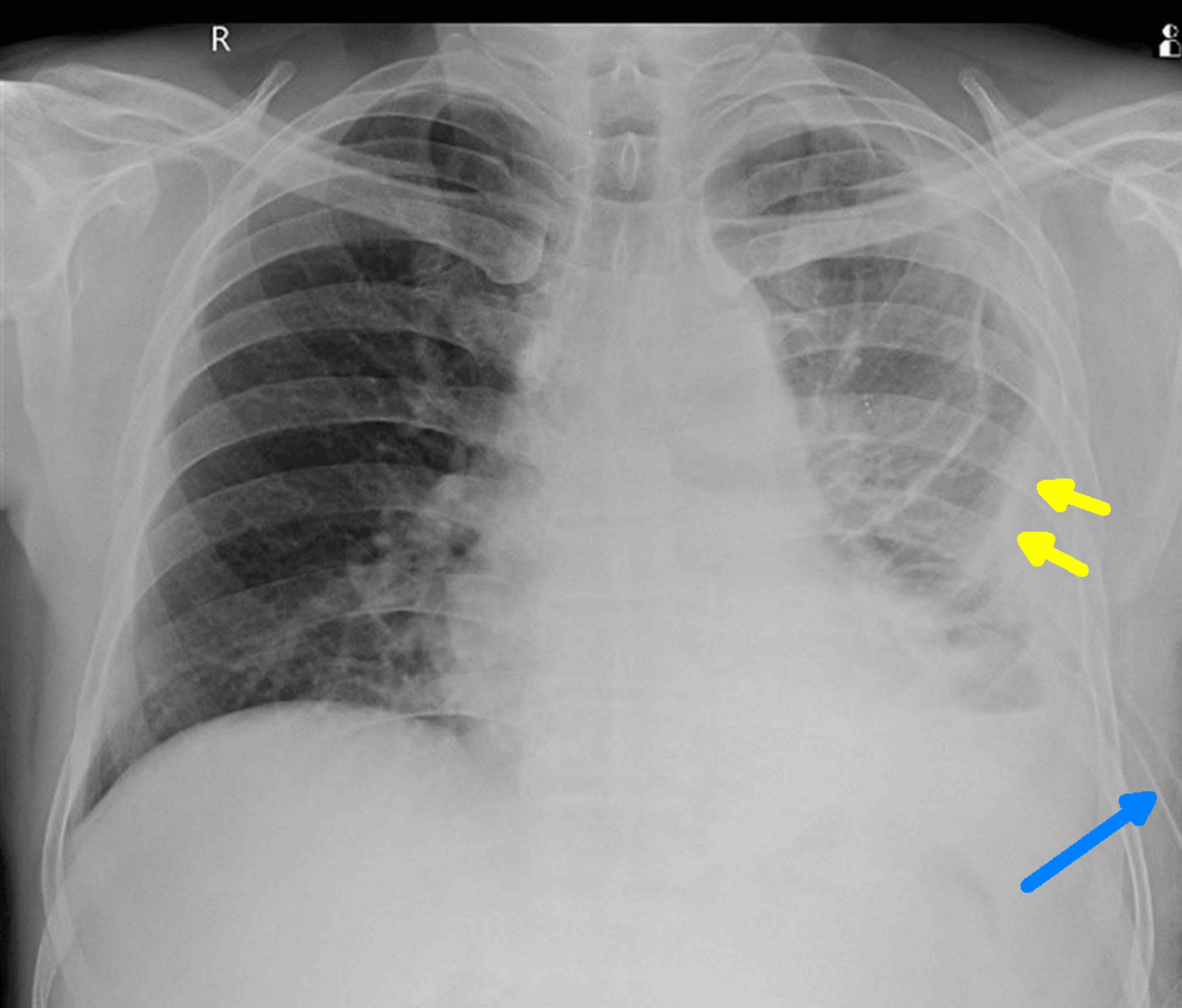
Chest X-ray post-thoracocentesis showing pleural thickening (yellow arrows) and intercostal drain in situ (blue arrow)
